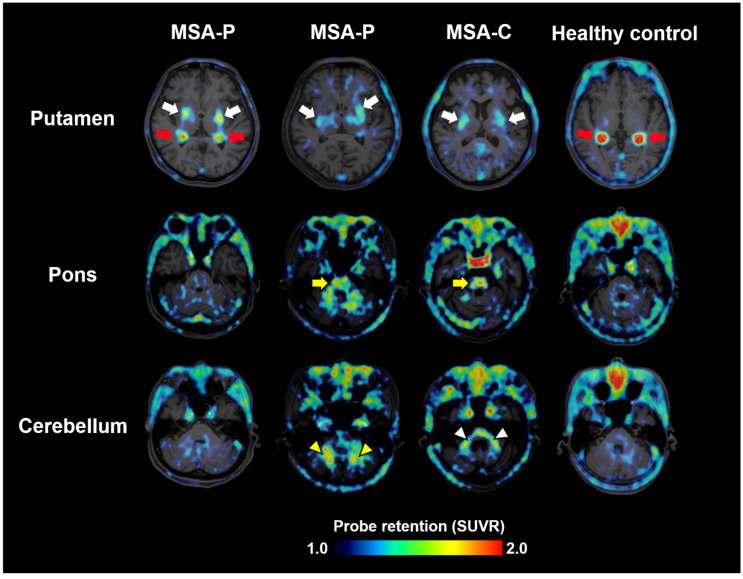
α-synuclein PET images acquired with ^18^F-SPAL-T-06 in healthy controls and cases with MSA-P and MSA-C. Red arrows indicate non-specific radioactivity accumulations in the choroid plexus. The probe captures pathologies in the putamen (white arrows), pons (yellow arrows), cerebellar peduncles (white arrowheads), and deep cerebellar white matter (yellow arrowheads). SUVR, standardized uptake ratio at 100–120 min after tracer injection. Modified from Ref. [18].
